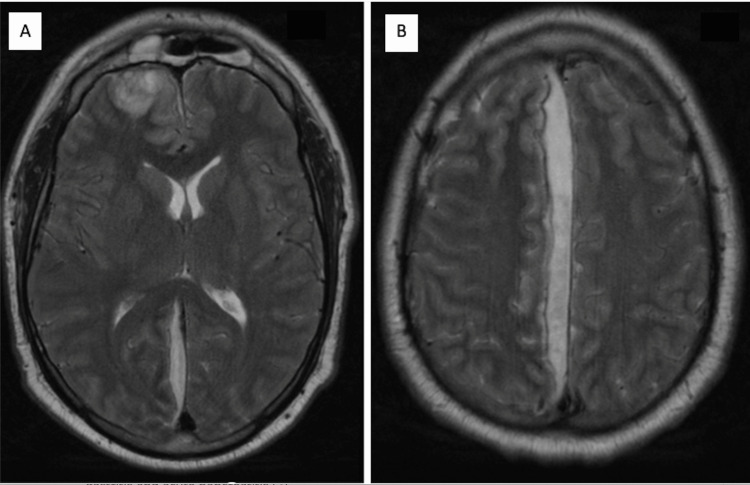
Brain MRI with IV contrast showing right frontal lobe lesion, which can be infectious and/or neoplastic in nature (A), and subdural fluid collection along the falx towards the right midline extending into the right tentorium (B)
